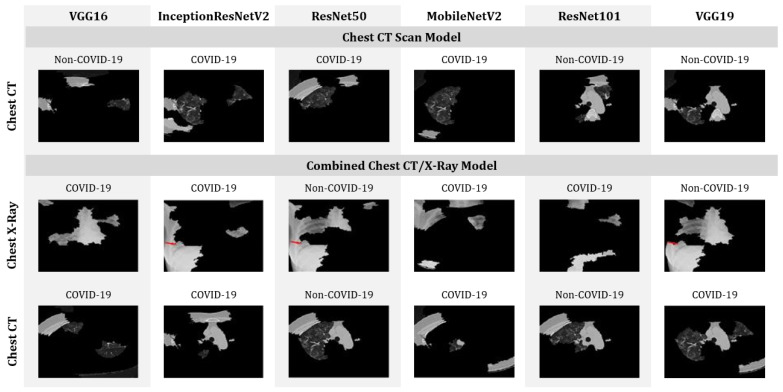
Top four features that enabled the identification of COVID-19 patients from CT-scan-only and mixed datasets.
